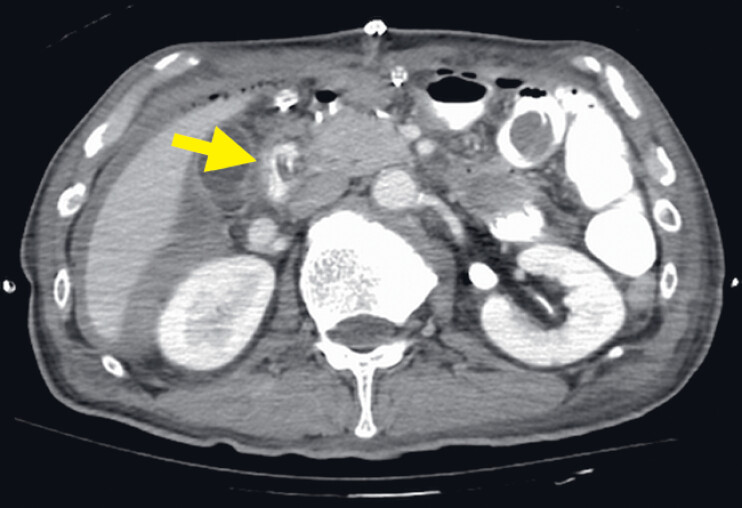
Contrast-enhanced computed tomography showing extravasation from the gastroduodenal artery (yellow arrowheads).
